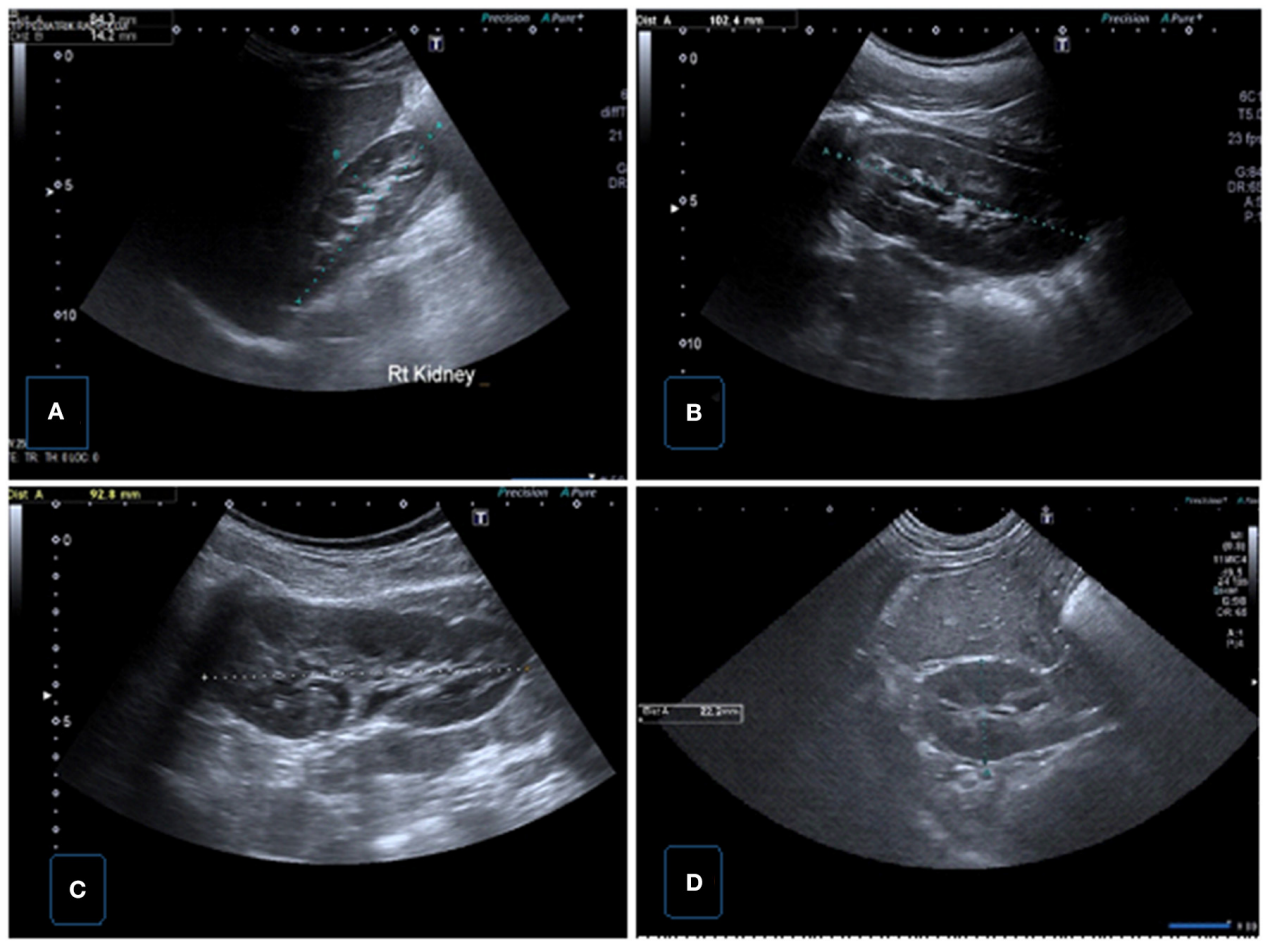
Normal renal sonographic images obtained with convex probes. (A) Longitudinal US image of the right kidney demonstrating renal length and parenchymal thickness in supine position. (B) Longitudinal US image of the left kidney demonstrating renal length in supine position. (C) Longitudinal US image of the left kidney demonstrating renal length in prone position. (D) Transverse US image of the right kidney showing renal AP size.
